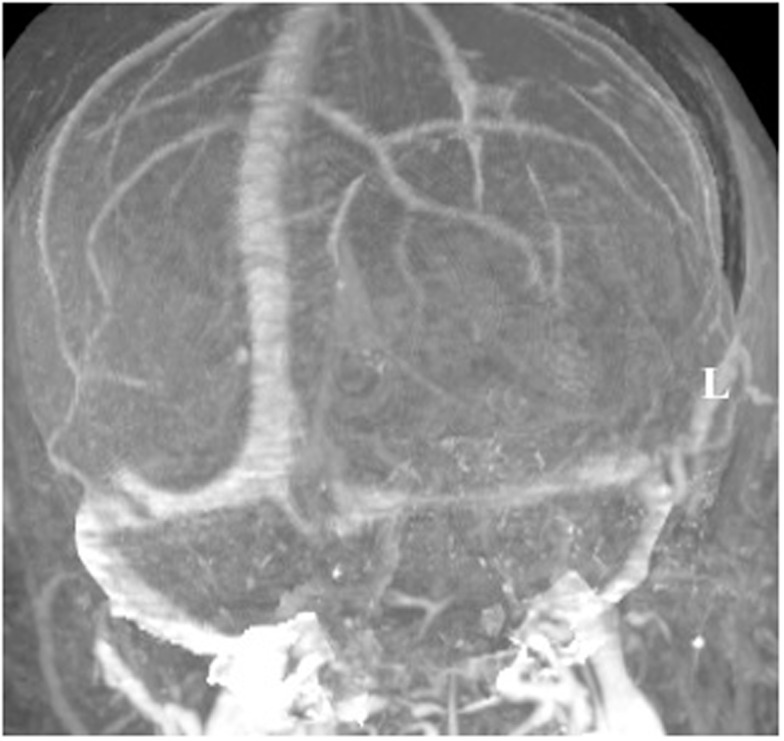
MRI venogram. An MRI venogram demonstrated that the left transverse sinus was atretic and the right transverse sinus was dominant.
